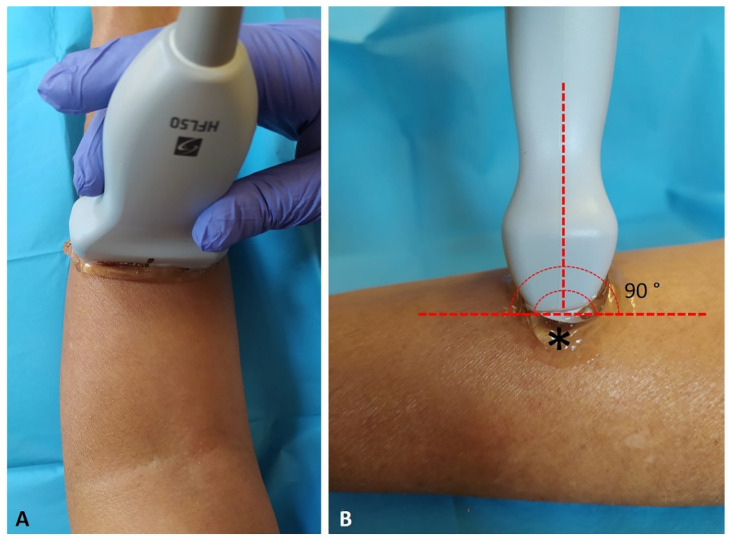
The probe position during the ultrasound (US) imaging assessment of the superficial fascia. (A): the anterior region of the forearm (Ant 1); (B) for adequate scans and to reduce surface pressure on the skin, the ultra-sonographer used suitable amounts of gel (*), and the US beam was kept perpendicular to the fascial layers.
